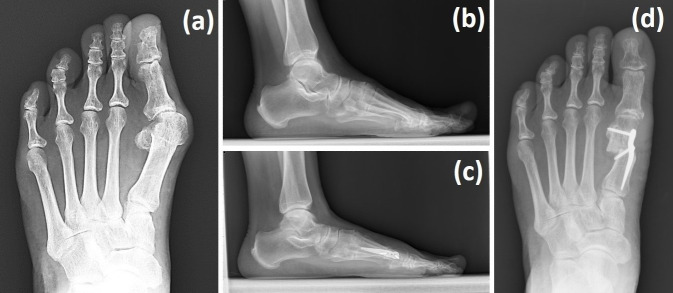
HV revision surgery performed using the Spear plate with Wilson osteotomy combined with a distal soft tissue procedure. The left foot of a 63-year-old male patient with HV recurrence was corrected, as shown in the above weight-bearing radiographs. (a,b) Preoperative radiographs and (c,d) 3-month-postoperative radiographs.
